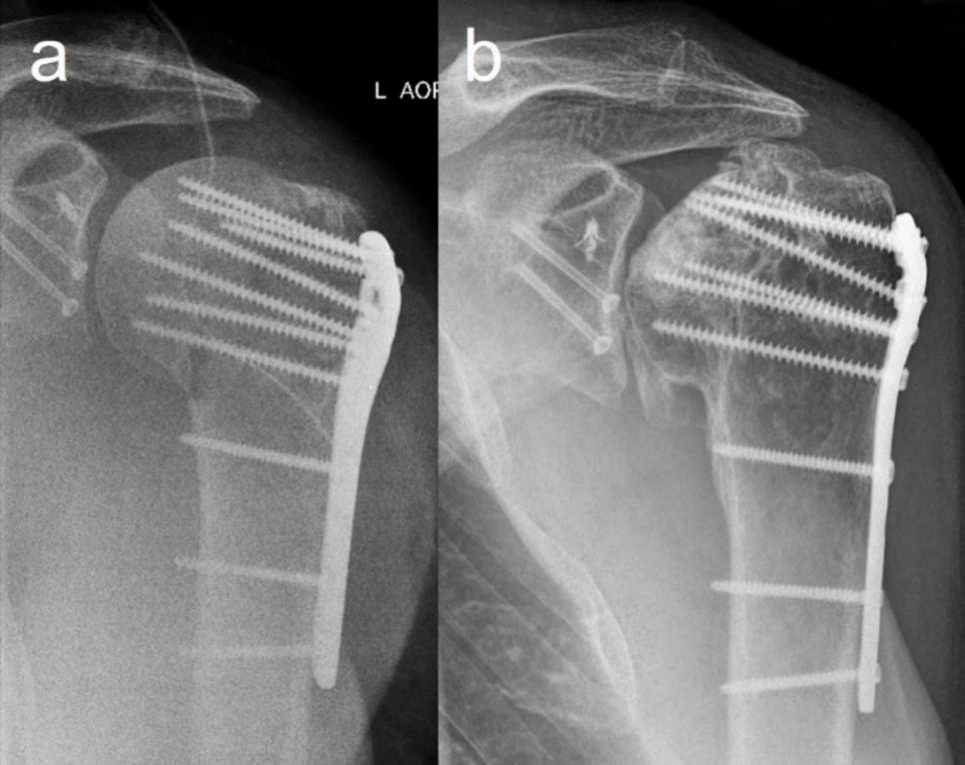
59 year old male with avascular necrosis of the humeral head 6 years after ORIF of both lesions of the glenohumeral combination fracture (a + b, a.p.-radiograph) with still moderate clinical function
